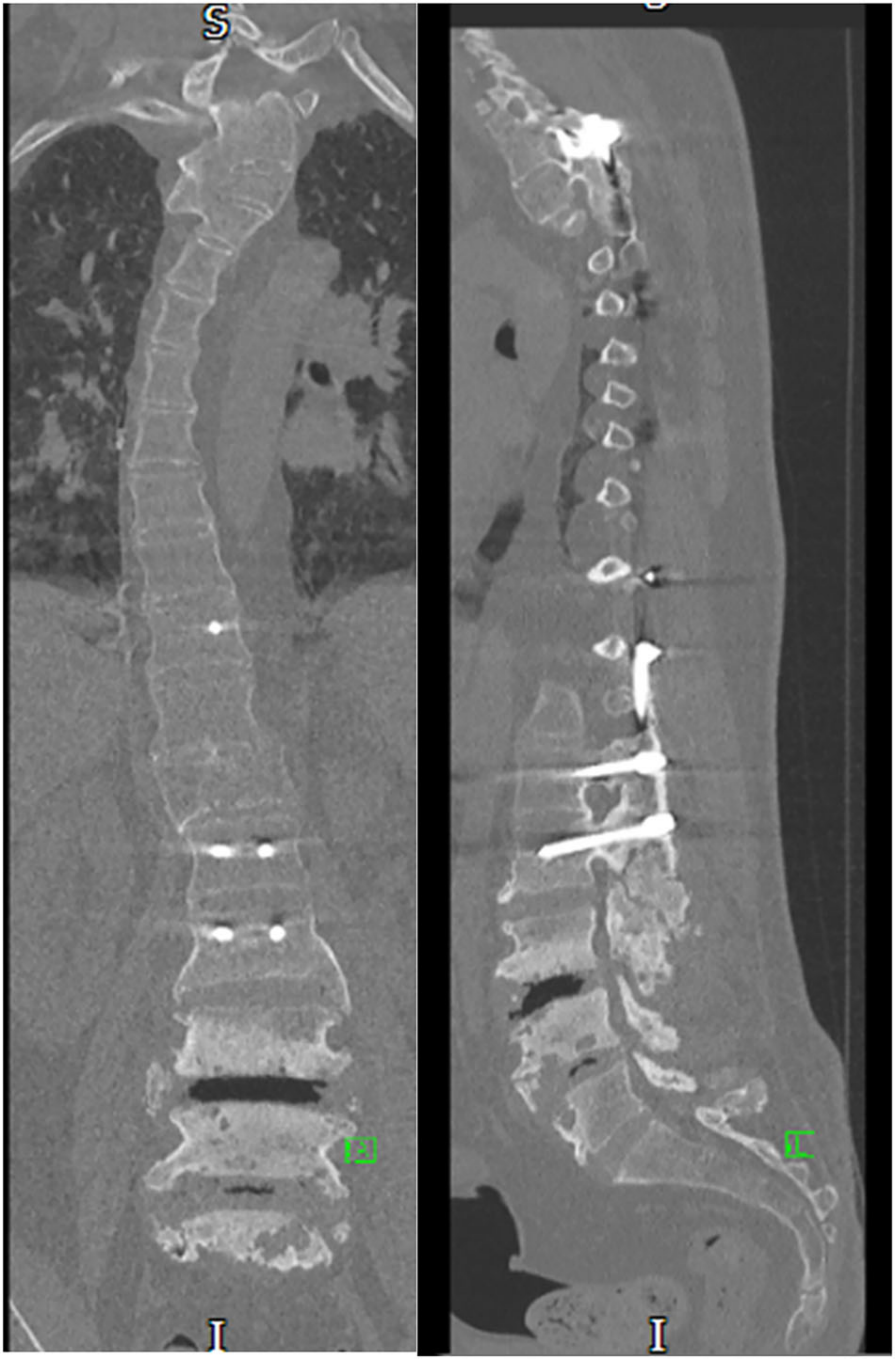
Coronal and sagittal view of the thoracic and lumbar spine CT: posterior rod and screw fixation noted from T2–L2. There is impression of solid fusion through these levels. Vacuum phenomenon and bony overgrowth noted at L3/L4.
